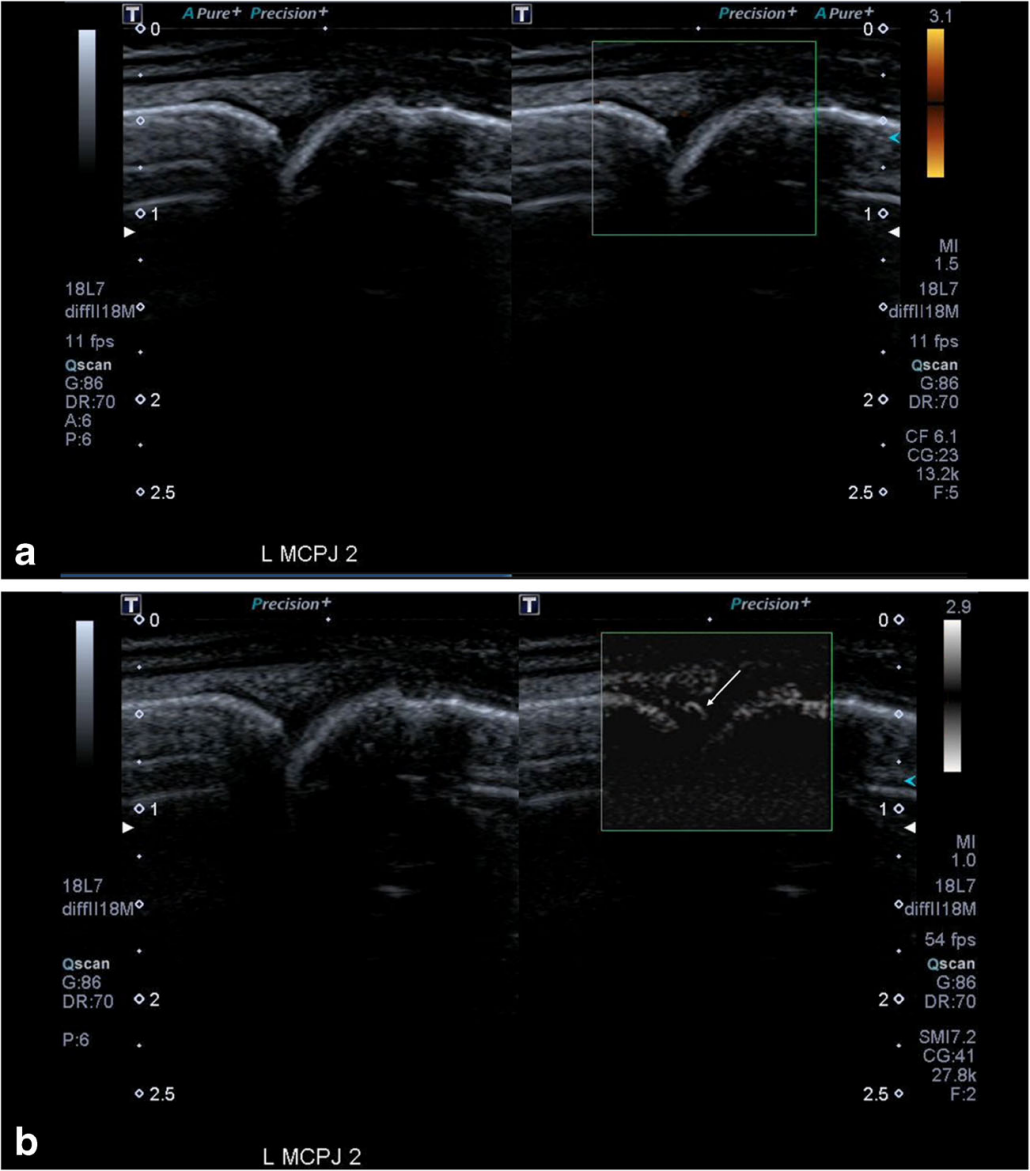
(a) These images show a relatively normal appearing joint on the grey-scale image of the metacarpophalangeal joint (MCPJ) of the left index finger, which was tender in this patient with rheumatoid arthritis. There is also no signal detected on Power Doppler ultrasound (PDUS). (b) The corresponding paired Superb Microvascular Imaging (SMI) images show that there is clear neovascularity seen within the joint (arrows) indicating active inflammation. This image highlights the resolution and flow within very small vessels that can be detected with SMI
